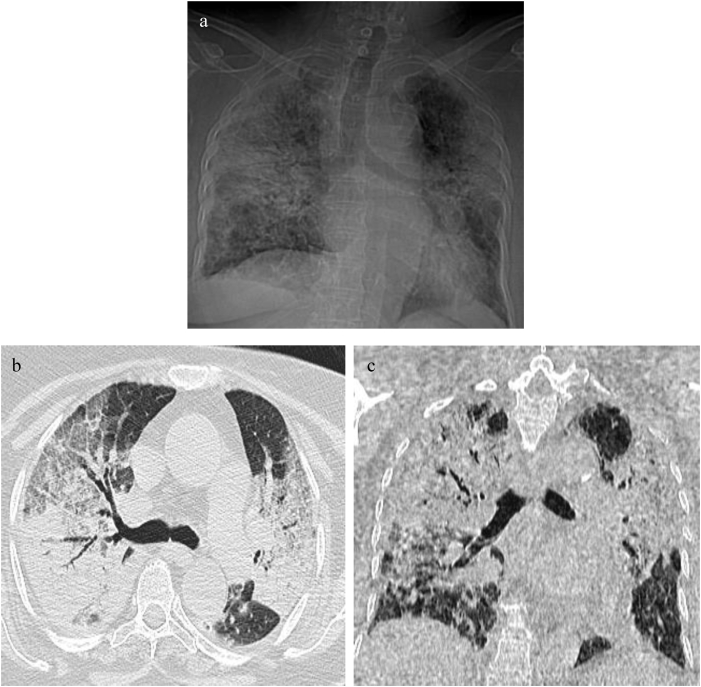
A 40 year-old-patient with a history of ten days of fever and coughing presented to the emergency department with an 86% O2 saturation and a 32/min respiratory rate. Frontal chest radiography (a) revealed extensive multilobar consolidations. Chest CT axial (b) and coronal (c) images showed typical severe peak-stage Covid-19 pneumonia imaging features with extensive ground glass opacities, crazy paving pattern, multilobar consolidations and bronchiectasis.
